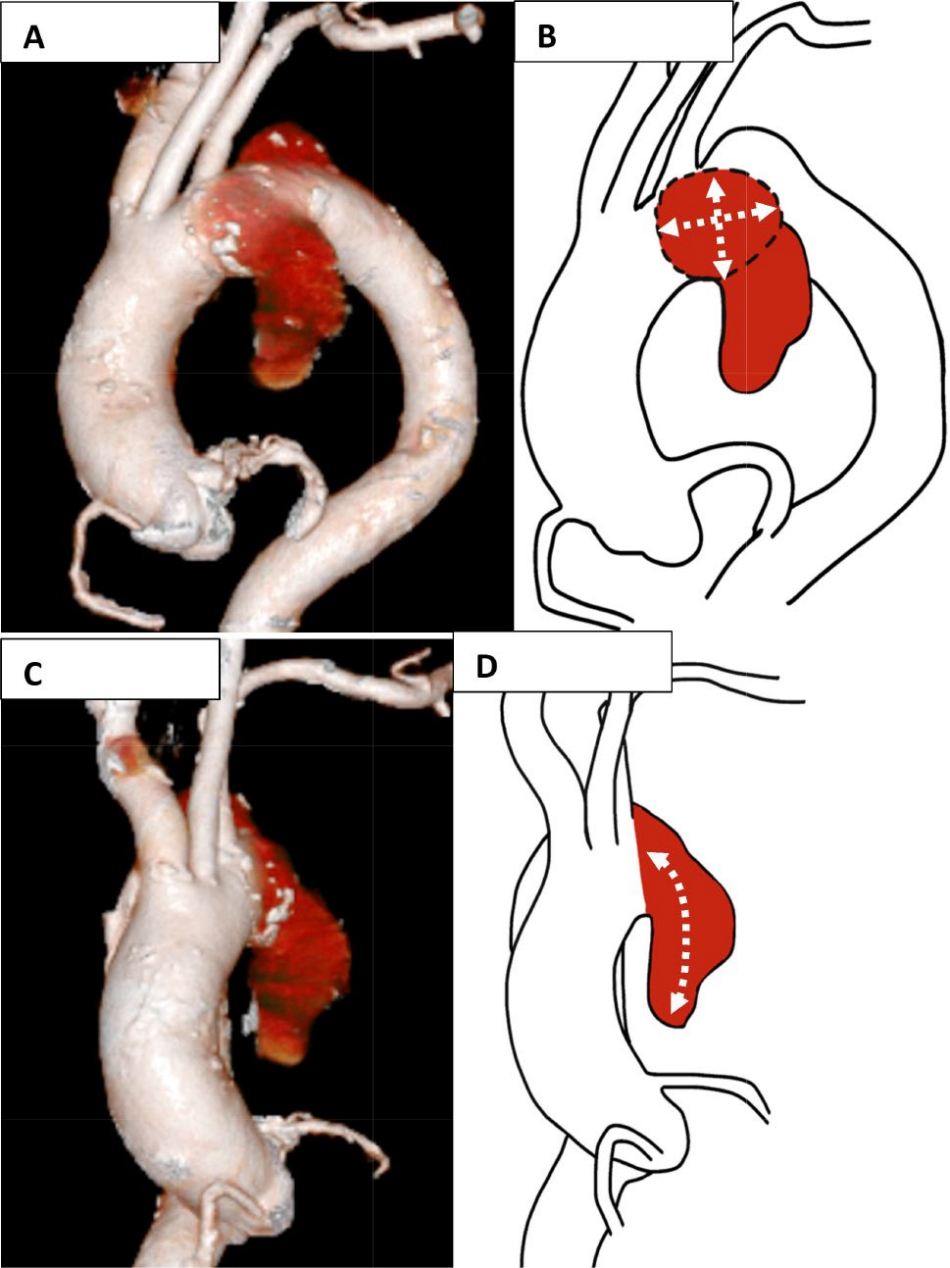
Preoperative 3D computed tomography. A, B: Front view. Saccular aneurysmal (Red color) width was 42 × 55 mm (White dashed arrowed). C, D: Left side view. Saccular aneurysmal neck was 84 mm (White dashed arrowed)
